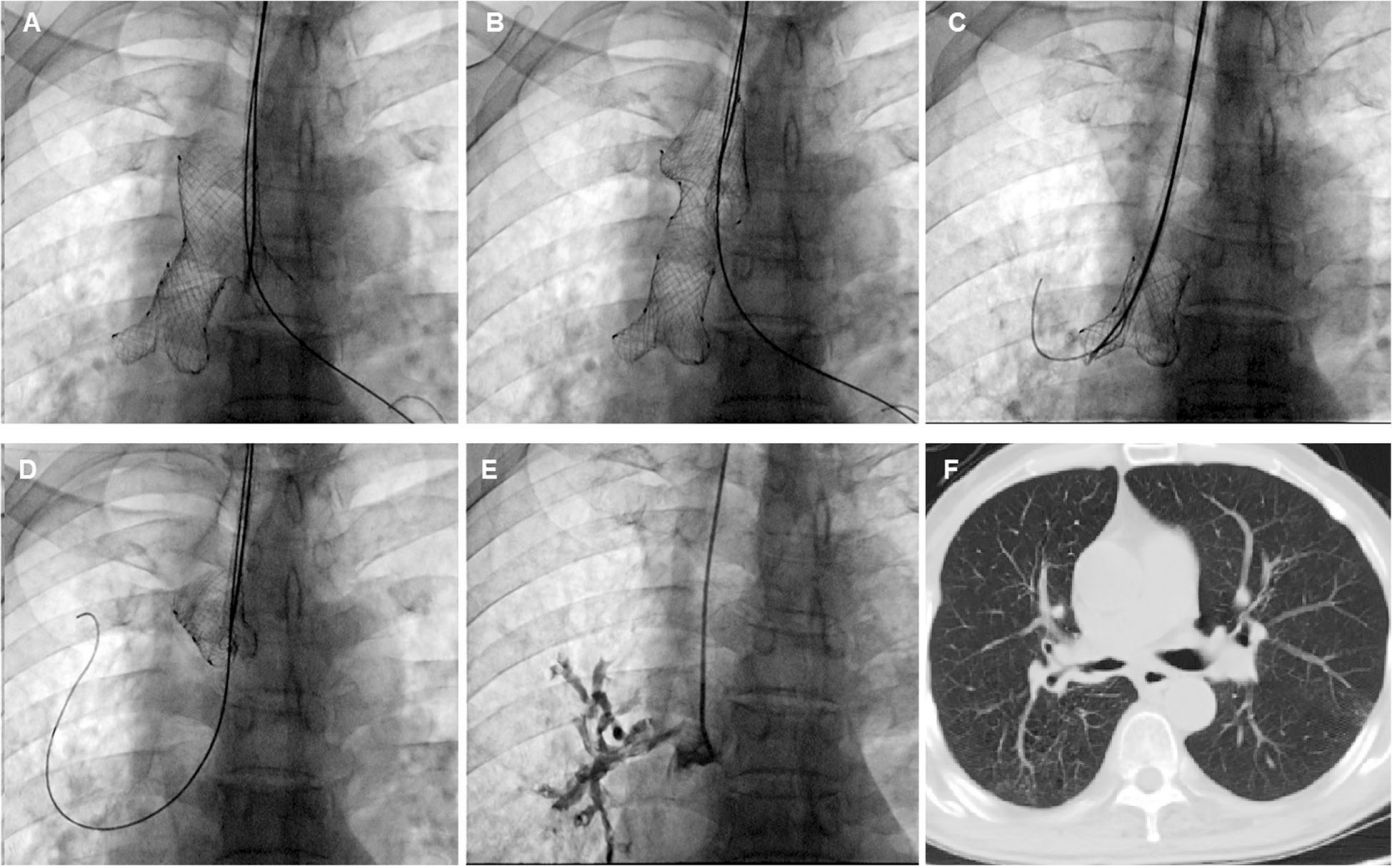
Stent removal after successful treatment. a The tip of the hook was placed next to the end of the large Y stent. b Metal Y stent was withdrawn from the airway wall. c The tip of the hook was placed next to the end of the small Y stent. d Small Y stent was withdrawn from the airway wall. e Radiography was performed again to confirm the seal of fistula immediately after stent removal. f Chest SCT showed the sealing of bronchopleural fistula and the disappearance of residual chest cavity
